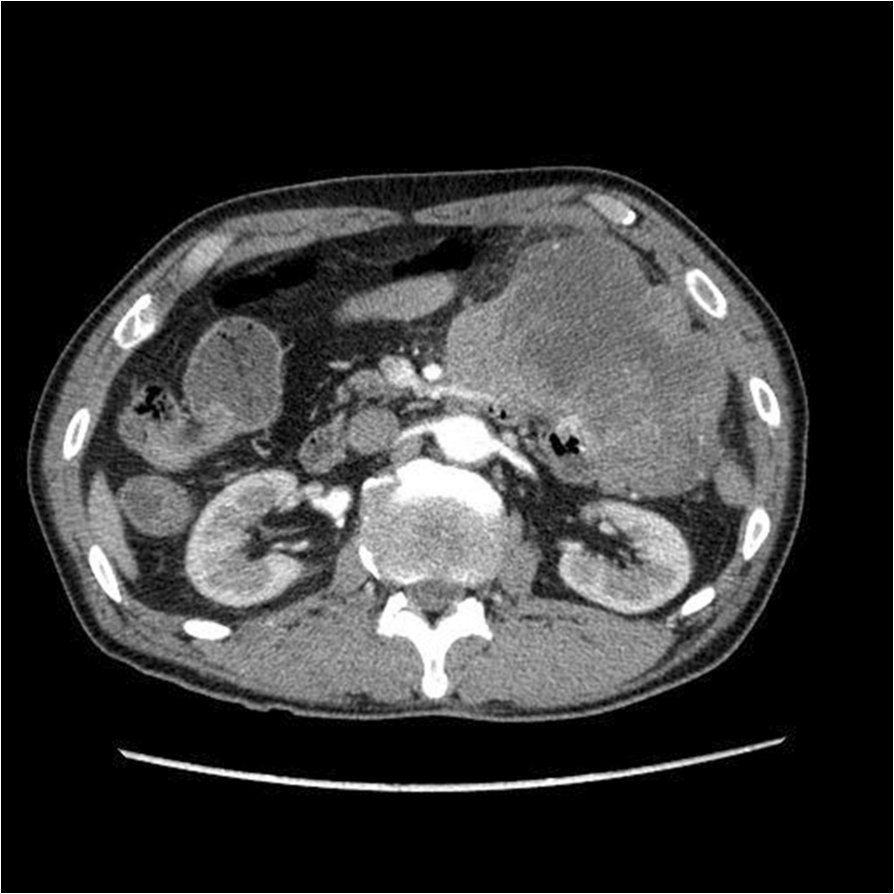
An abdominal enhanced computed tomography scan, revealing a 10 × 10-cm solid tumor with low-density area within, suggesting necrosis or abscess.
